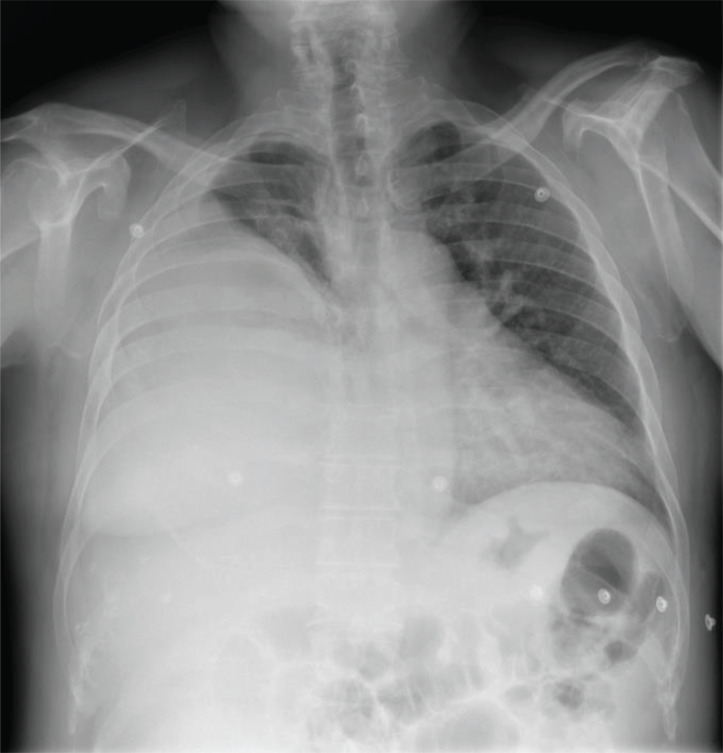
Chest X-ray showing a well-demarcated homogeneous space-occupying lesion. Trachea is central. The right principal bronchus is not well defined. Cardiomediastinal shadow is lightly pushed on left side.
